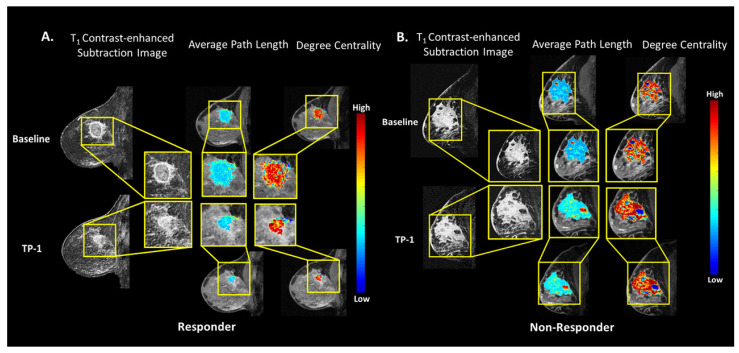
Examples of the breast MRI validation dataset in a responder and nonresponder patients after NACT. (A) Breast MRI in a typical responder patient that received NACT. The patient’s lesion decreased in size after NACT treatment. Shown are the overlays of the TCF metrics of degree centrality and average path length maps with decreases in the degree centrality and average path lengths. (B) Breast MRI in a nonresponder patient that received NACT with an increase in the patient’s lesion size after NACT. Shown are the overlays of the TCF metrics of degree centrality and average path length maps with increases in the degree centrality and average path lengths.
